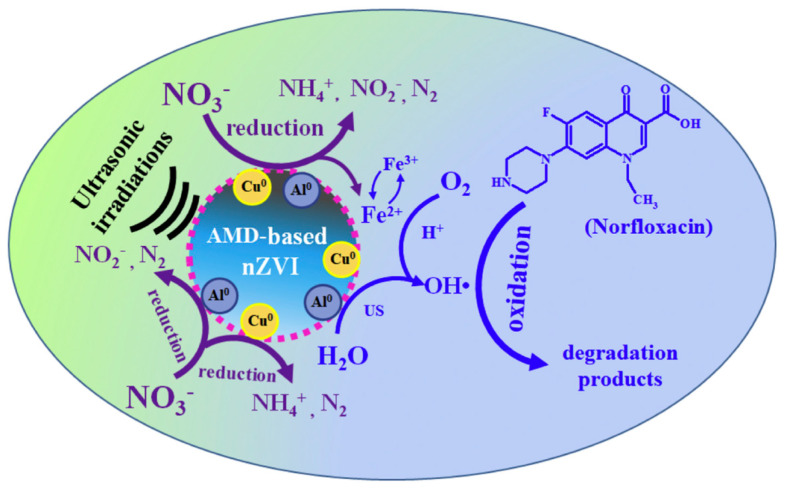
Possible mechanism for the simultaneous removal of nitrate and norfloxacin by acid mine drainage-based nZVI with ultrasonic irradiation. Reproduced with permission from [73]. Copyright Elsevier Inc., 2019.
